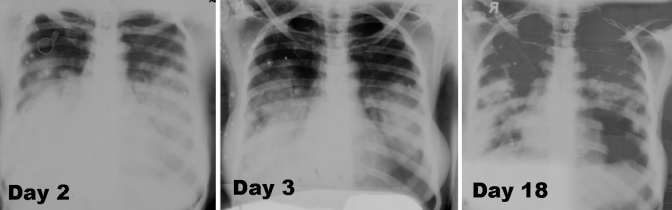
Three serial frontal chest radiographs from surviving caregiver B2 with primary pneumonic plague obtained on illness days 2, 3, and 18 showing bilateral lower lung zone predominant airspace disease associated with bilateral (right > left) pleural effusions. The radiographs have artifacts related to hand-dipping of the films, which account for multiple densities that move between images and the areas of apparent lucency.
